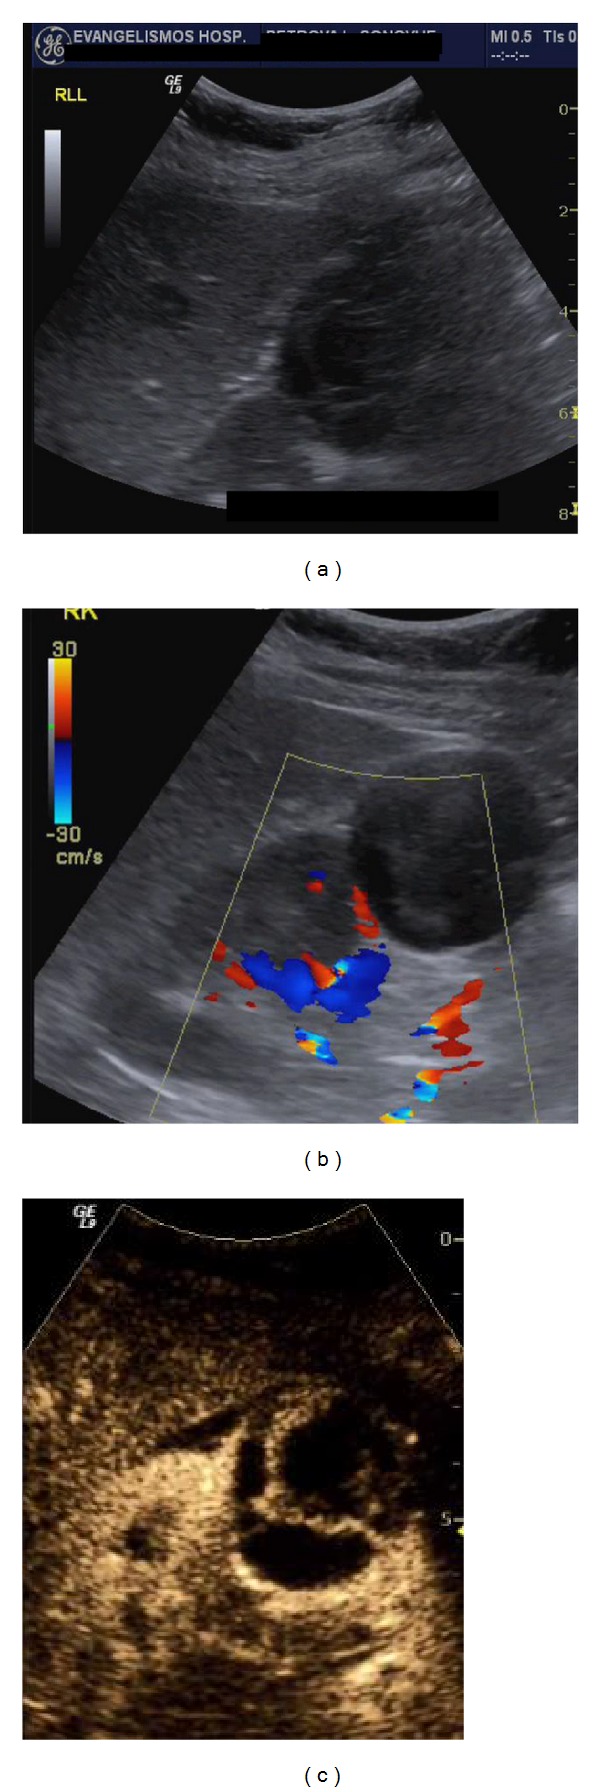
Bosniak III cyst: a mixed echogenicity cortical lesion is noted in the right kidney on B-mode US (a). Colour Doppler (b) does not reveal increased vascularity inside the lesion. However, CEUS (c) shows rich enhancement in the cyst's septa. This finding classifies the lesion as a Bosniak III renal cyst.
